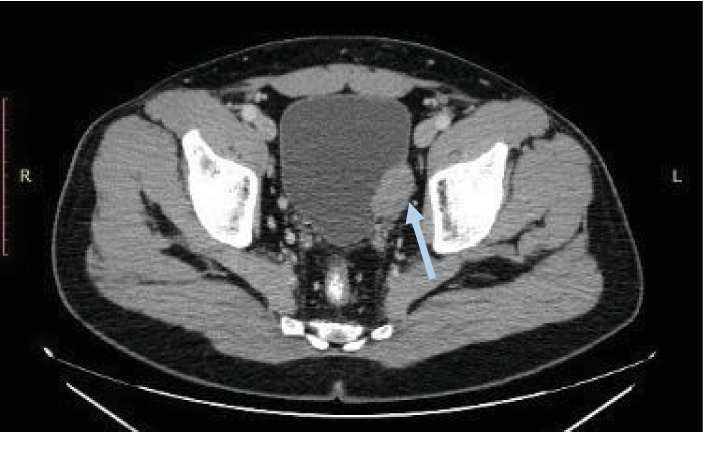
Bladder leiomyoma (grey arrow). Computed tomography, low abdomen. Horizontal view.
